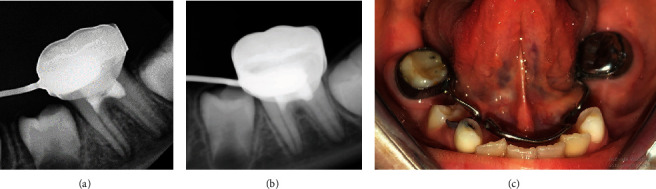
Follow-up images: (a) 6 months periapical radiograph, (b) 12 months periapical radiograph, and (c) 12 months final intraoral image.
